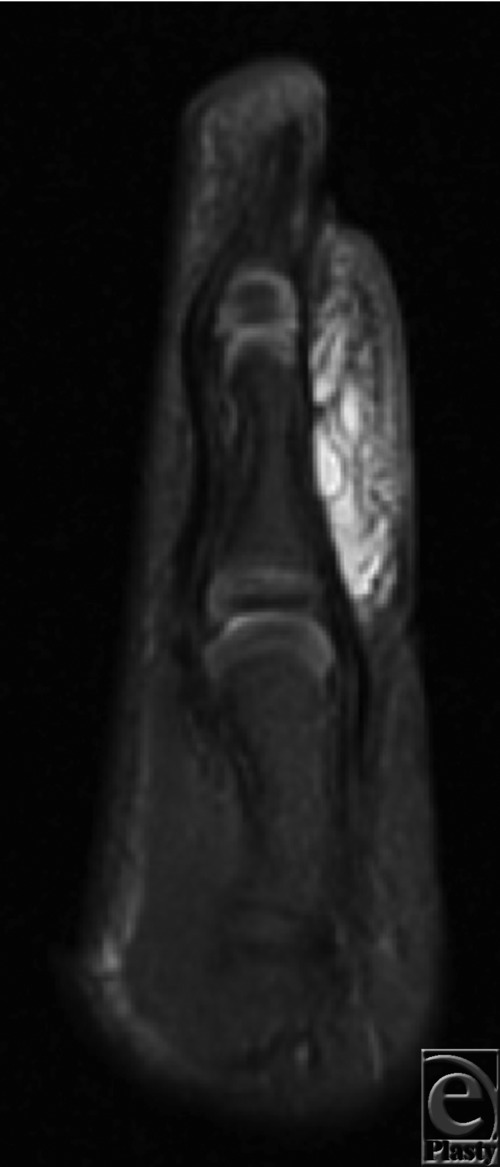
Magnetic resonance image of thumb mass.
